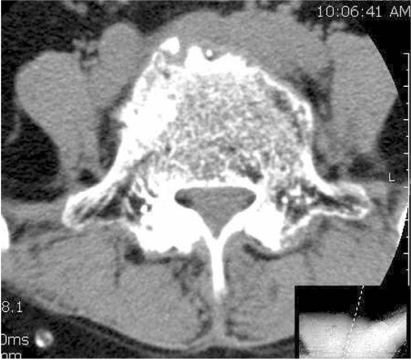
CT scan shows thecal sac compression without the destruction of bone or paravertebral absecess.
